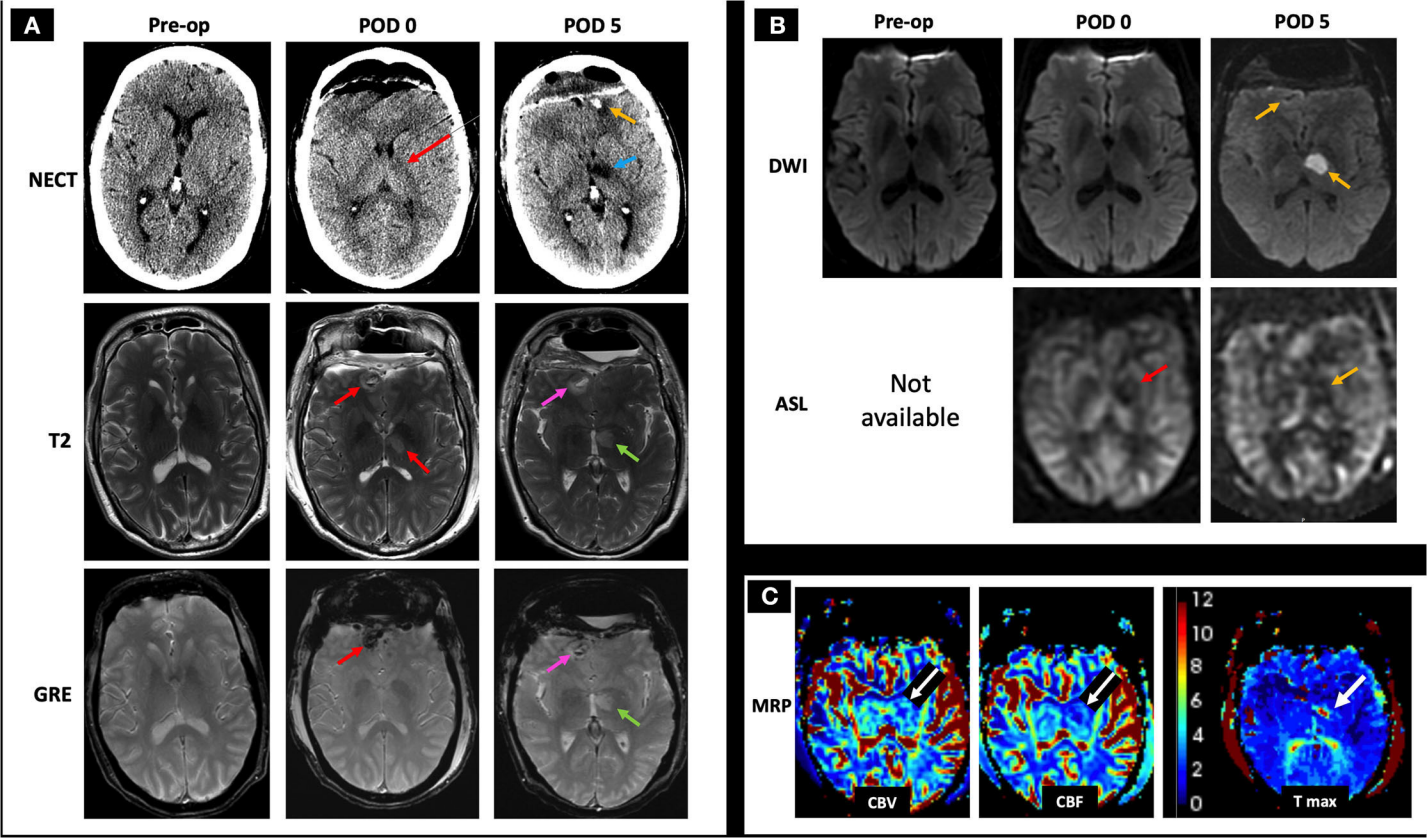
Case 5: A 57-year-old male with history of pituitary macroadenoma status post subtotal resection with subsequent bilateral frontal craniotomy for residual tumor removal 1 week prior to presentation developed acute altered mental status in the immediate postoperative period. The normal preoperative NECT, T2, GRE, and DWI images are included for comparison (A, left column; B, left column). Immediate postoperative (POD 0) NECT shows pneumocephalus with subtle hypodensity of the thalamus and posterior limb of left internal capsule (A, middle image in the top row, red arrow), and immediate postoperative MRI shows small bifrontal T2 hyperintense lesions with mild surrounding edema and associated GRE hypointensity (A, middle column, red arrows), as well as decreased ASL signal on qualitative perfusion-weighted ASL images without abnormal DWI signal (B, middle column, red arrow indicating hypoperfusion on ASL image), suspicious for hemorrhagic CVT. On follow-up 4 days later (POD 5), NECT showed a more conspicuous left thalamic hypodensity (A, right column, blue arrow) in addition to previously seen bifrontal hemorrhages (shown at the left frontal pole with a yellow arrow), concerning for infarcts. C-MRI showed T2 hyperintense signal and GRE hypointensity associated with the bifrontal hemorrhagic infarcts (A, right column, pink arrows point to the right frontal hemorrhage) and left thalamic infarct (A, right column, green arrows). Follow-up MRI (POD 5) showed diffusion restriction and decreased ASL signal at the left thalamus (B, left column, yellow arrows) with associated decreased CBV and CBF as well as increased Tmax (C, white arrows), consistent with CVT. Given the history of prolonged clamp time across the frontal lobe and the non-arterial distribution of these lesions, the bilateral frontal parenchymal hemorrhages are suggestive of iatrogenic CVT. AMS, altered mental status; CBF, cerebral blood flow; CBV, cerebral blood volume; C-MRI, contrast-enhanced MRI; ICV, internal cerebral veins; NECT, non-contrast CT; Tmax, time-to-maximum.
